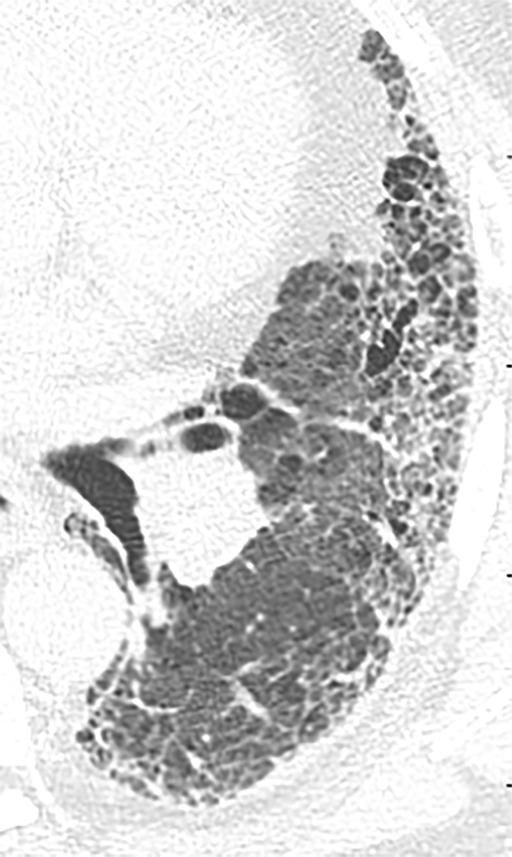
Acute exacerbation of idiopathic pulmonary fibrosis. (a) Zoomed view of axial CT demonstrates a probable usual interstitial pneumonia pattern in a patient with idiopathic pulmonary fibrosis. (b) Zoomed view of axial CT obtained after rapid deterioration in symptoms demonstrates CT findings of acute exacerbation with progression of reticular abnormality and marked new superimposed ground-glass opacity. Other causes of airspace disease including infection were clinically excluded. (c) Zoomed view of axial CT obtained after resolution of acute exacerbation confirms that the background fibrosis has substantially progressed.
